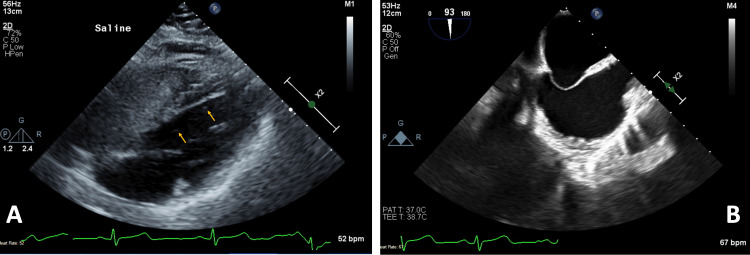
TEE demonstrating (A) crossover of bubbles (yellow arrows) on an agitated saline study from the right heart to the left heart within three cardiac cycles, indicative of an intracardiac shunt; and (B) a bicaval view of the interatrial septal aneurysm. TEE = transesophageal echocardiogram
